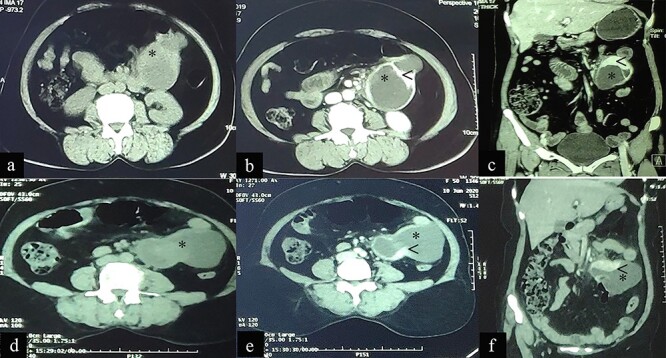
Case 2: CT imaging of Extragastrointestinal stromal tumor. (a) Non-contrast axial; (b, c) contrast enhanced axial and coronal reformatted CT images of abdomen demonstrates intraperitoneal cystic lesion (*) with eccentric enhancing solid component (<) abutting kidney and bowel. (d) Non-contrast axial; (e, f) contrast enhanced axial and coronal reformatted CT images of abdomen of same patient after 1-year interval follow up demonstrates increased size of the lesion (*).
